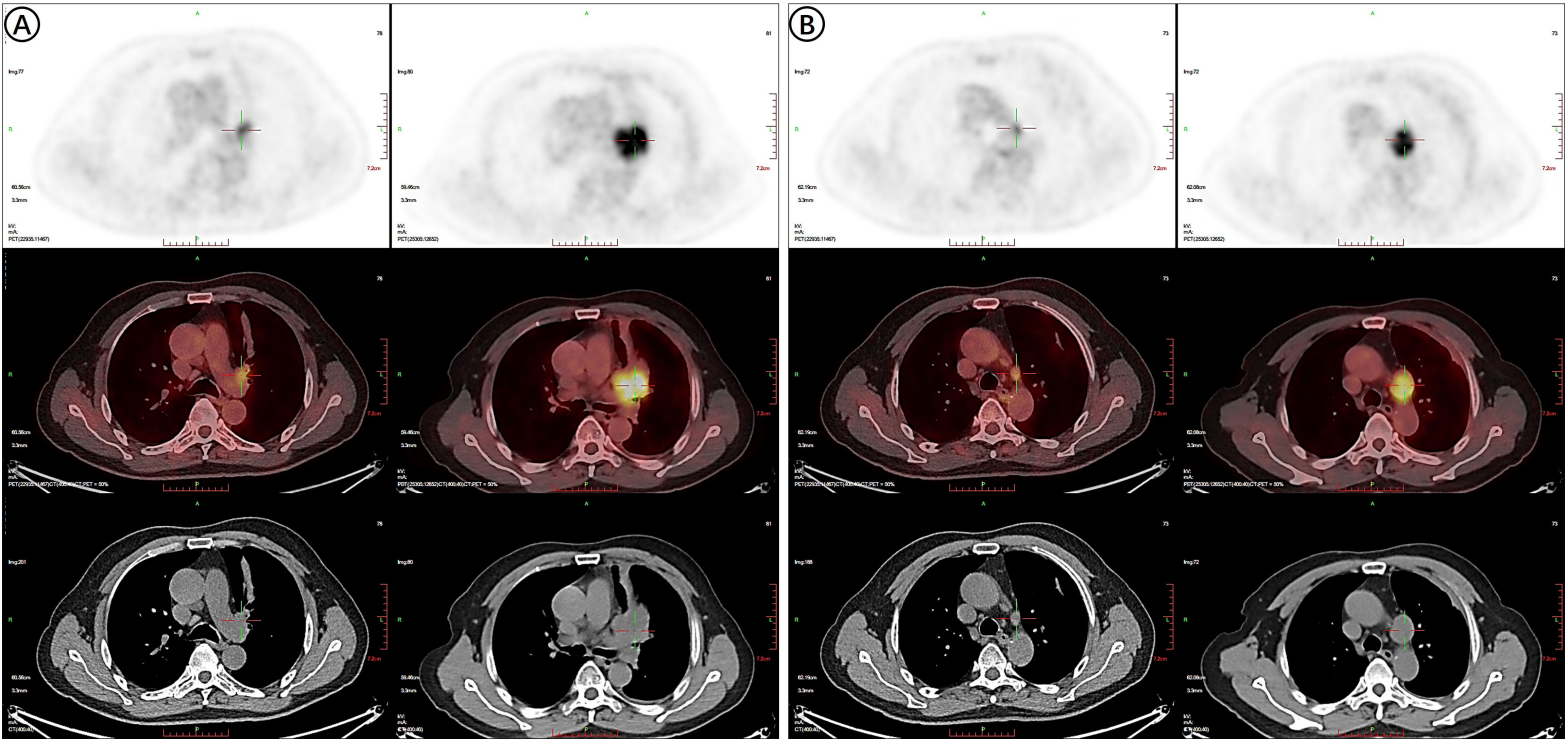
PET-CT findings of the pulmonary tumor in 2023 (A) and 2024 (B).
